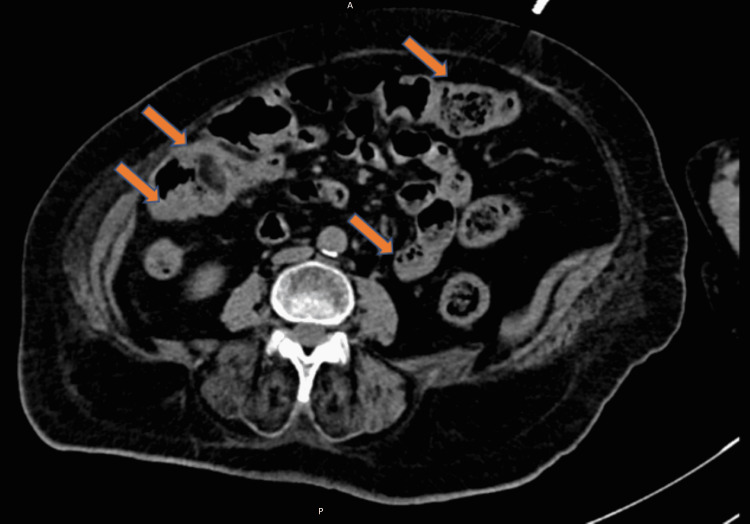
Computed tomography (CT) scan showing C. difficile colitis (arrows). A: anterior; P: posterior
